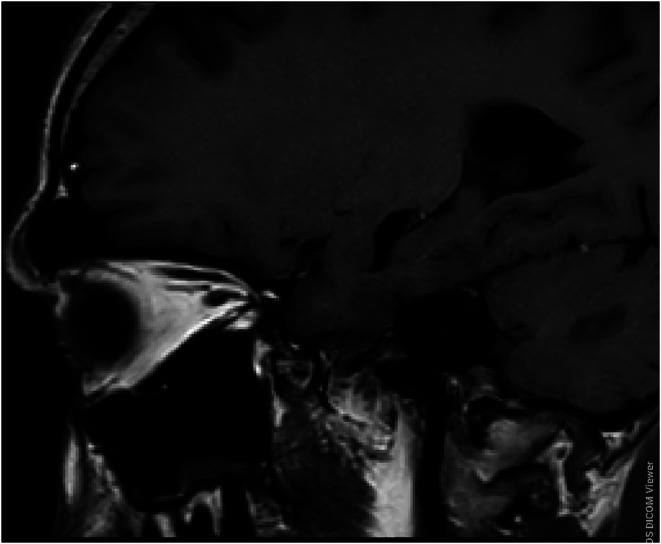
Sagittal pituitary magnetic resonance image demonstrating structural abnormalities of the hypothalamic–pituitary axis, including thinning of the pituitary stalk and a small anterior pituitary gland. The normal posterior pituitary bright spot is not identified.
